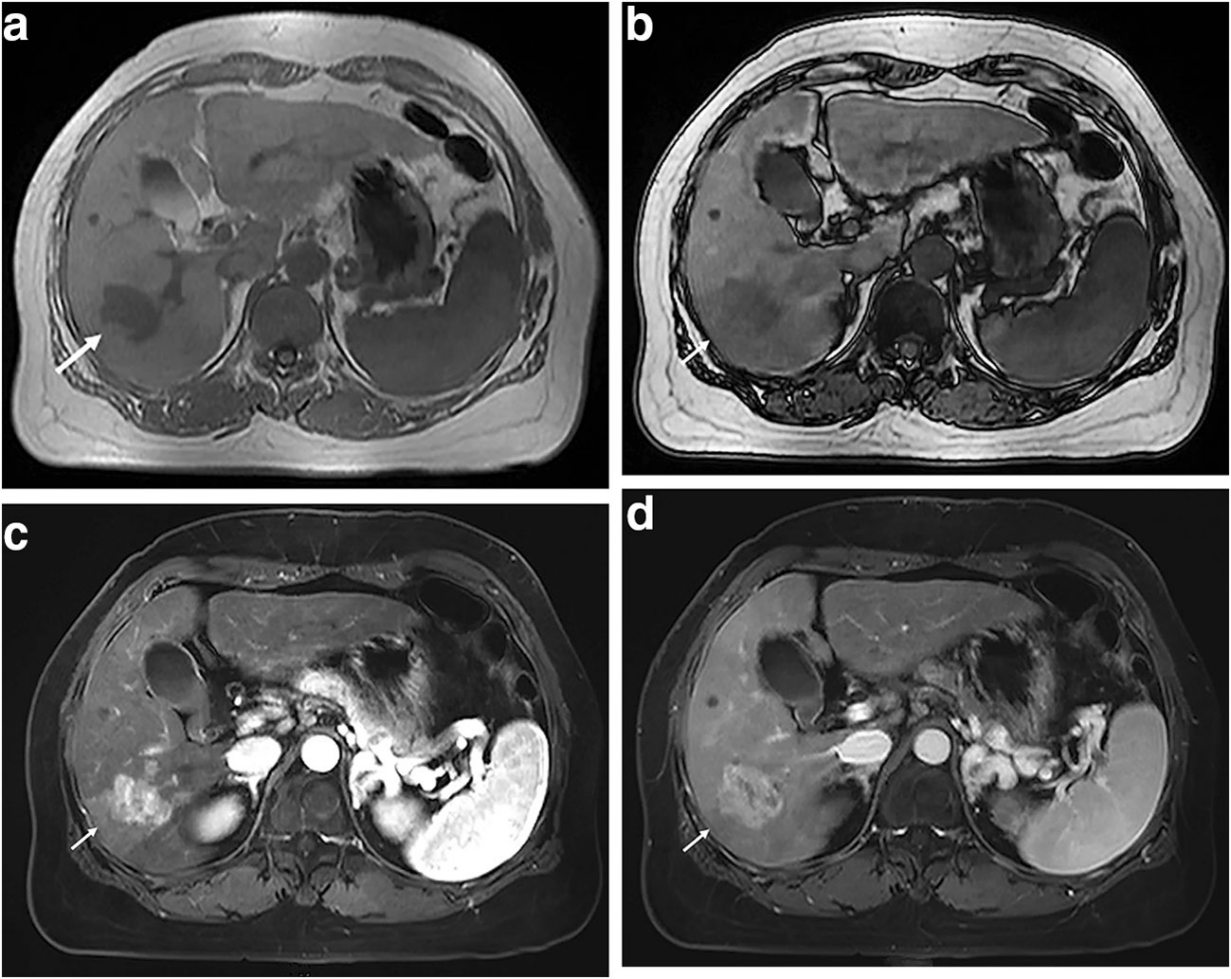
Images in a 58-year-old woman with cHCC-CCA with MVI; her serum level of AFP was 1885 ng/ml. a In-phase MR image shows a 3.0-cm hypointense irregular mass (arrow) in segment VI of the liver. b On the opposed-phase image, there was no obvious signal drop in the lesion (arrow), indicating the absence of an unambiguous fatty-containing lesion. c Axial arterial phase image shows a hypervascular mass with irregular peritumoral enhancement (arrow). d Portal venous phase image shows nonsmooth tumor margin and peritumoral slight hypointensity (arrow)
